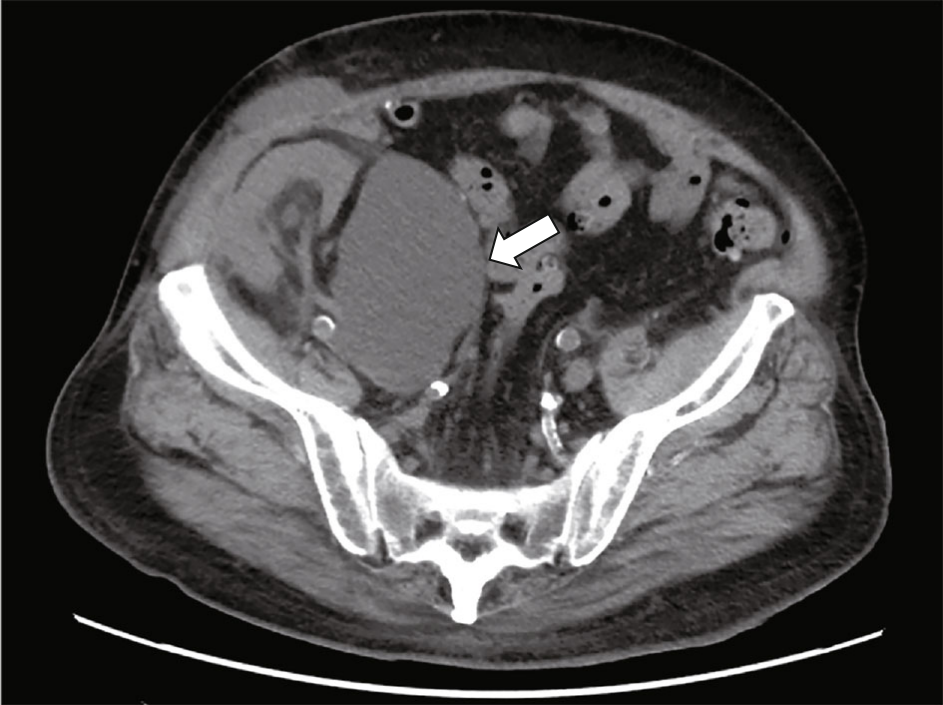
Computed tomography (CT). A lymphocele (16 cm × 8 cm × 6 cm) on the left side of the transplanted kidney in the right iliac fossa (white arrow) is detected.
